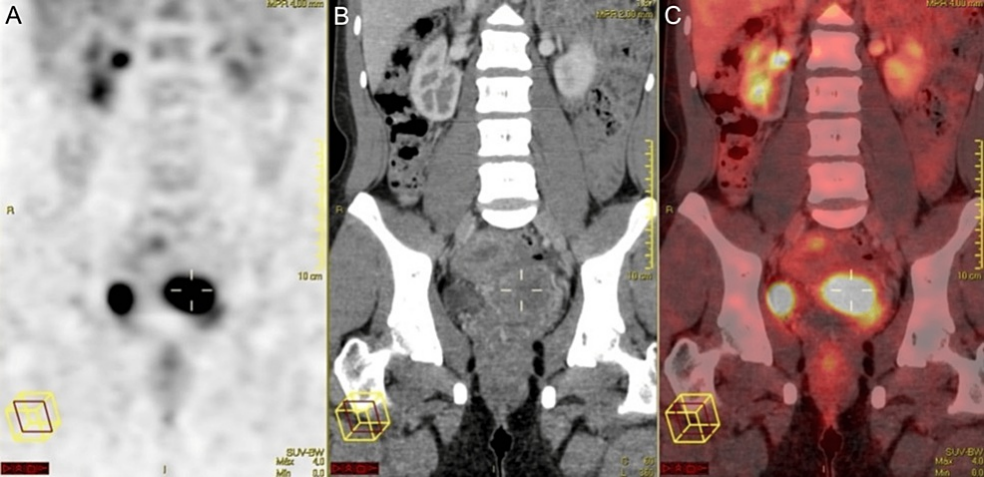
(A): Positron emission tomography scan (PET). (B): Computed tomography scan (CT). (C): Combined PET/CT scan.
